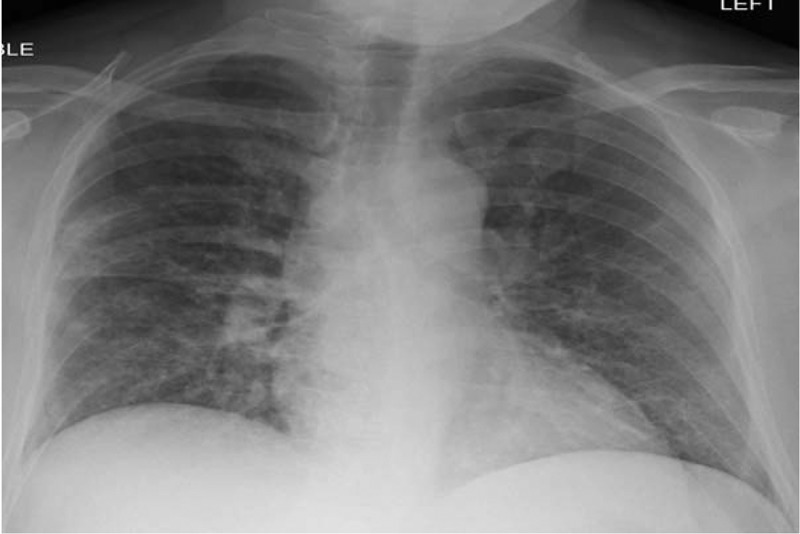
Chest radiograph done after discharge showed resolution of pulmonary infiltrates.
